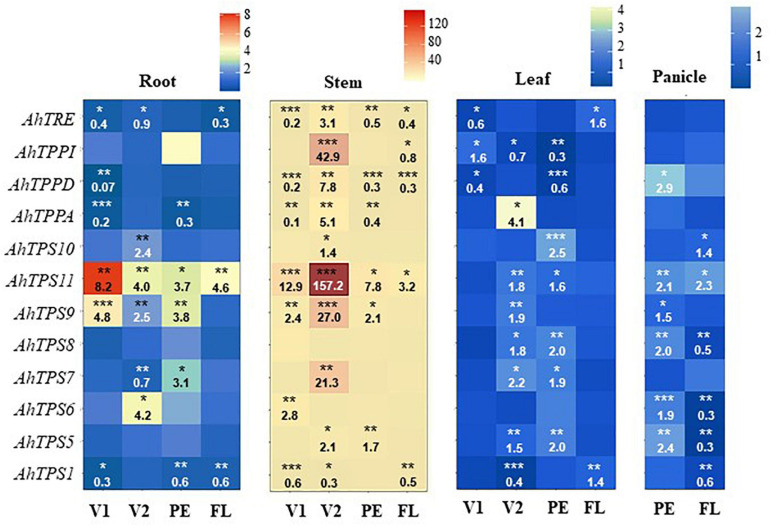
Changes in the expression of trehalose metabolism genes in defoliated *versus* non-defoliated *Amaranthus cruentus* plants. Data shown compare completely defoliated versus intact *A. cruentus* plants at four different developmental stages: vegetative 1 (V1), vegetative 2 (V2), panicle emergence (PE), and flowering (FL). RNA was extracted for each stage from the following tissues: roots, stems, leaves, and panicles. qRT-PCR was then performed for genes coding for enzymes involved in trehalose-6-phosphate (Tre6) synthesis (trehalose phosphate synthase, TPS class I: *AhTPS1*), signaling (TPS, class II: *AhTPS5-11*), Tre6P de-phosphorylation (trehalose phosphate phosphatase, TPP: *AhTPPA*, *D* and *I*), and trehalose degradation (trehalase, TRE, *AhTRE*). Relative expression was quantified based on the 2^–ΔΔ*CT*^ calculation (Livak and Schmittgen, 2001). C_T_ values for all genes were normalized to the C_T_ value of the *AhACT7* and *AhEF1a* housekeeping genes. Then, the changes in gene expression were calculated from four technical replicates. Asterisks indicate statistically significant differences with undefoliated controls: P* < 0.05, *P* < 0.01, or P* < 0.001 (*n* = 4) for a one-way ANOVA, Tukey HSD test. Data shown are representative of an experiment that was replicated twice. Changes are visualized using a different color code for each tissue, as indicated in the upper section of the figure.
